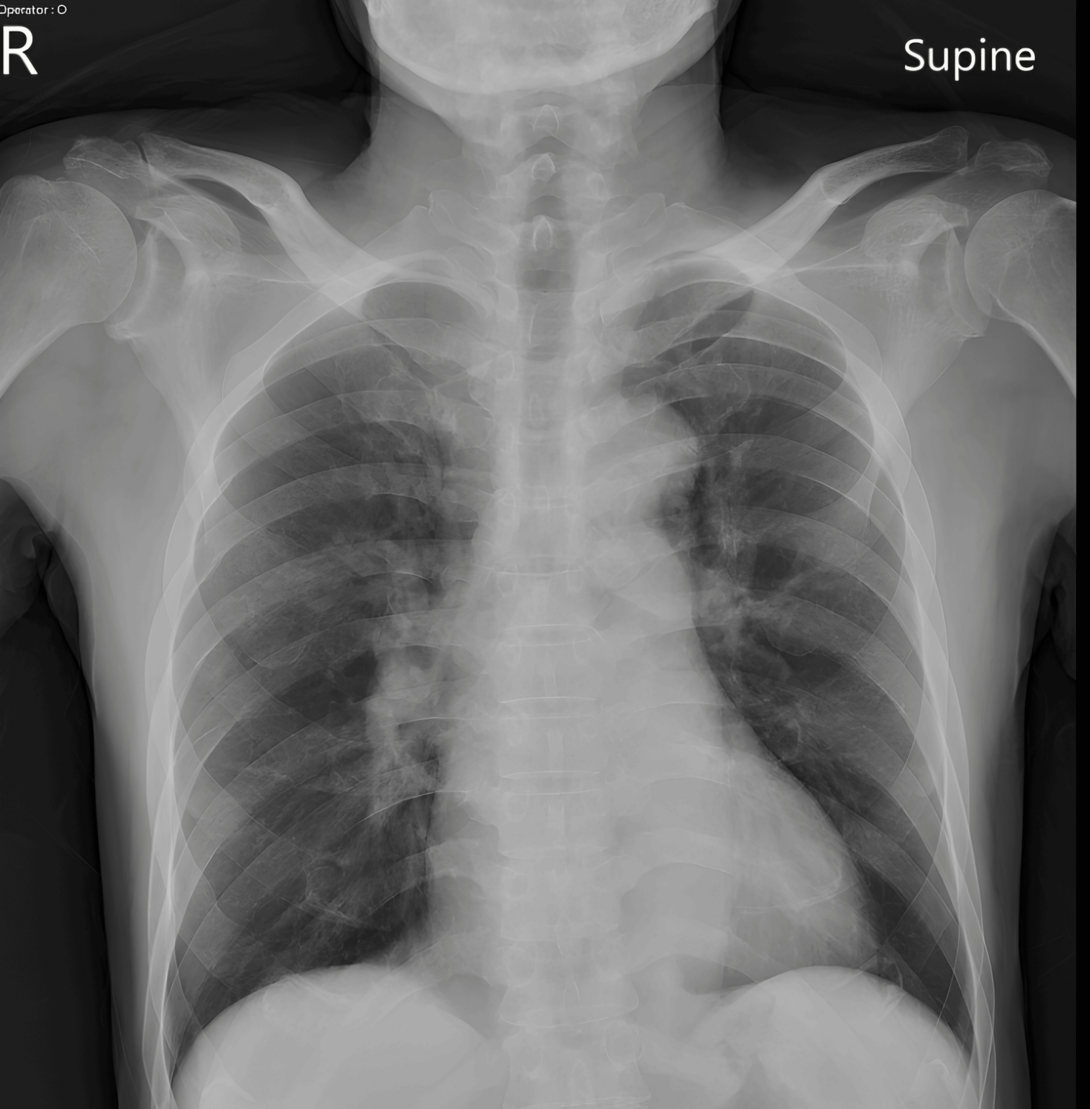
Chest radiograph on presentation The bilateral lung fields appear relatively clear without evidence of significant pulmonary edema, vascular congestion, or active consolidation. The cardiomediastinal silhouette and costophrenic angles are unremarkable.
